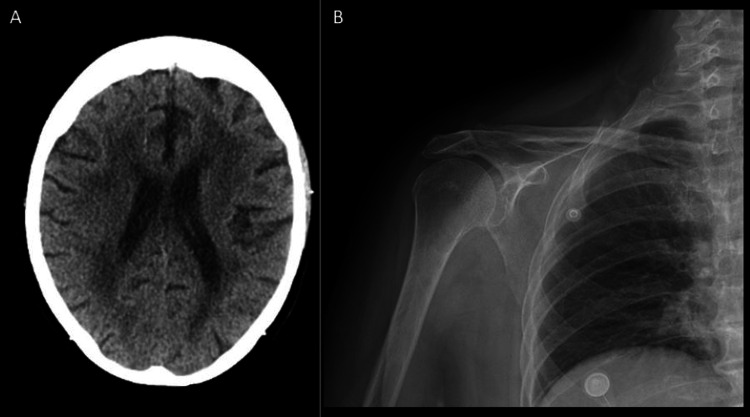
Radiologic imaging A) CT of the head revealed chronic findings without any acute intracranial abnormalities or neurovascular changes; B) X-ray of the right shoulder showed degenerative changes associated with mild osteoarthritis
